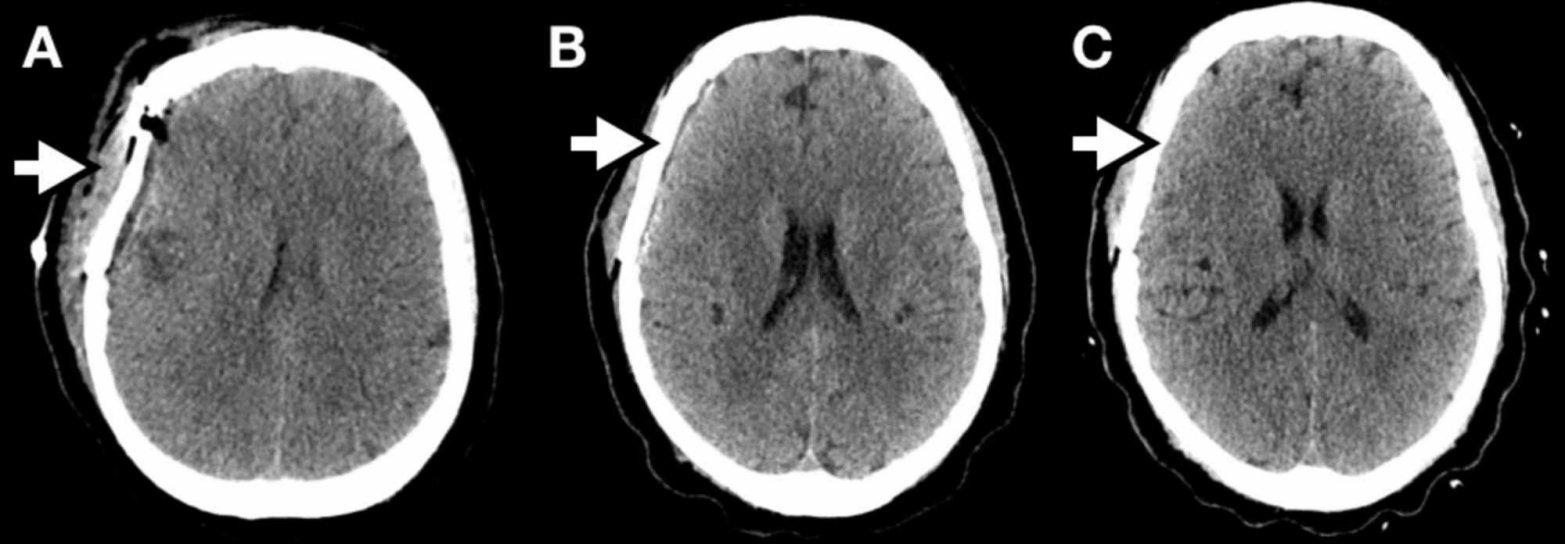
Post-operative computed tomography head images Surveillance images obtained one day (A), one month (B), and three months (C) post-operatively show gross total resection of the right frontal-temporal lesion. There are multiple known, persistence extra-axial lesions.
